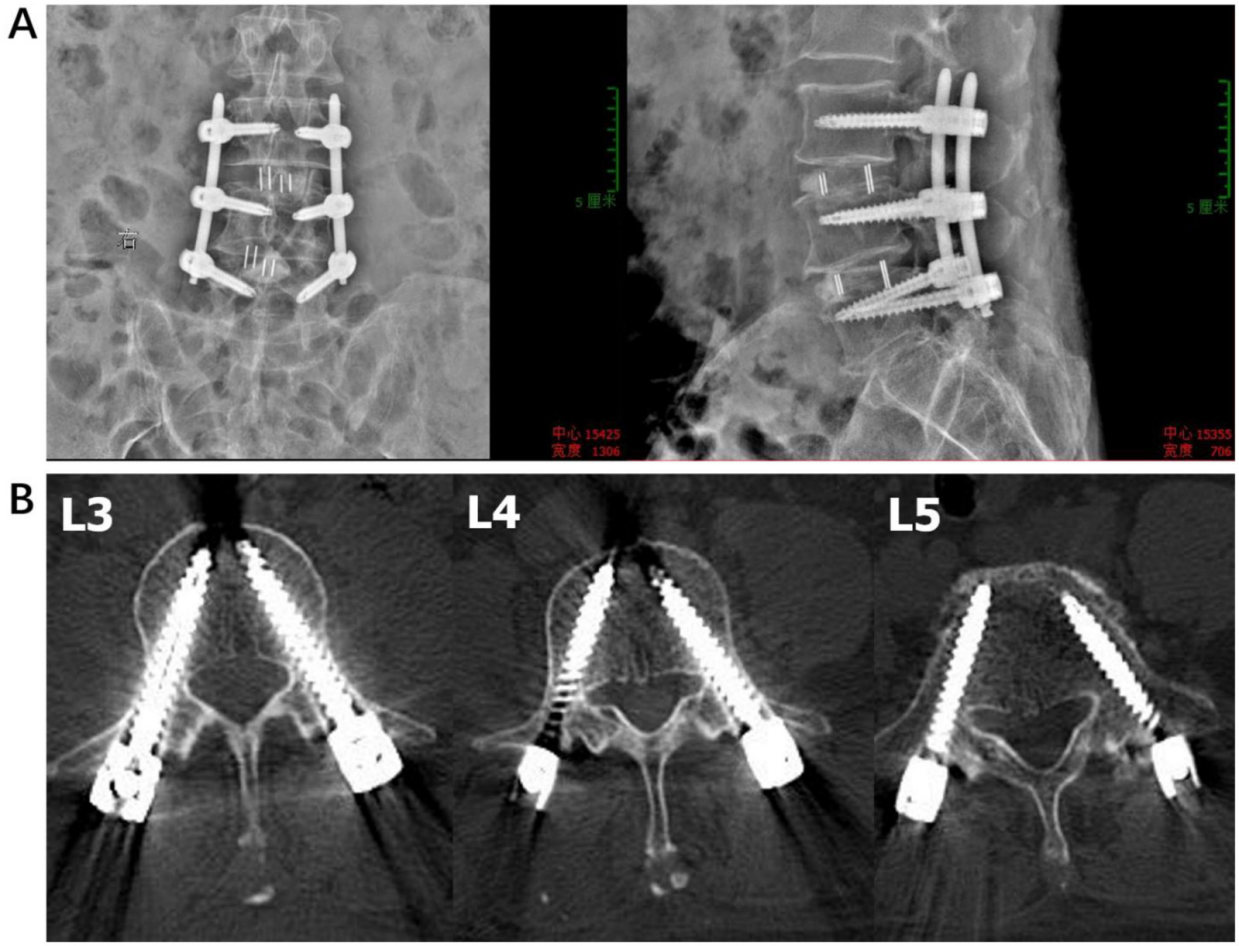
Postoperative imaging of a patient with lumbar disc herniation undergoing three-step fluoroscopic guided PPS surgery. (A) Anteroposterior and lateral x-ray views. (B) CT scan of the L3-L5 vertebrae, demonstrating satisfactory placement of the pedicle screws.
